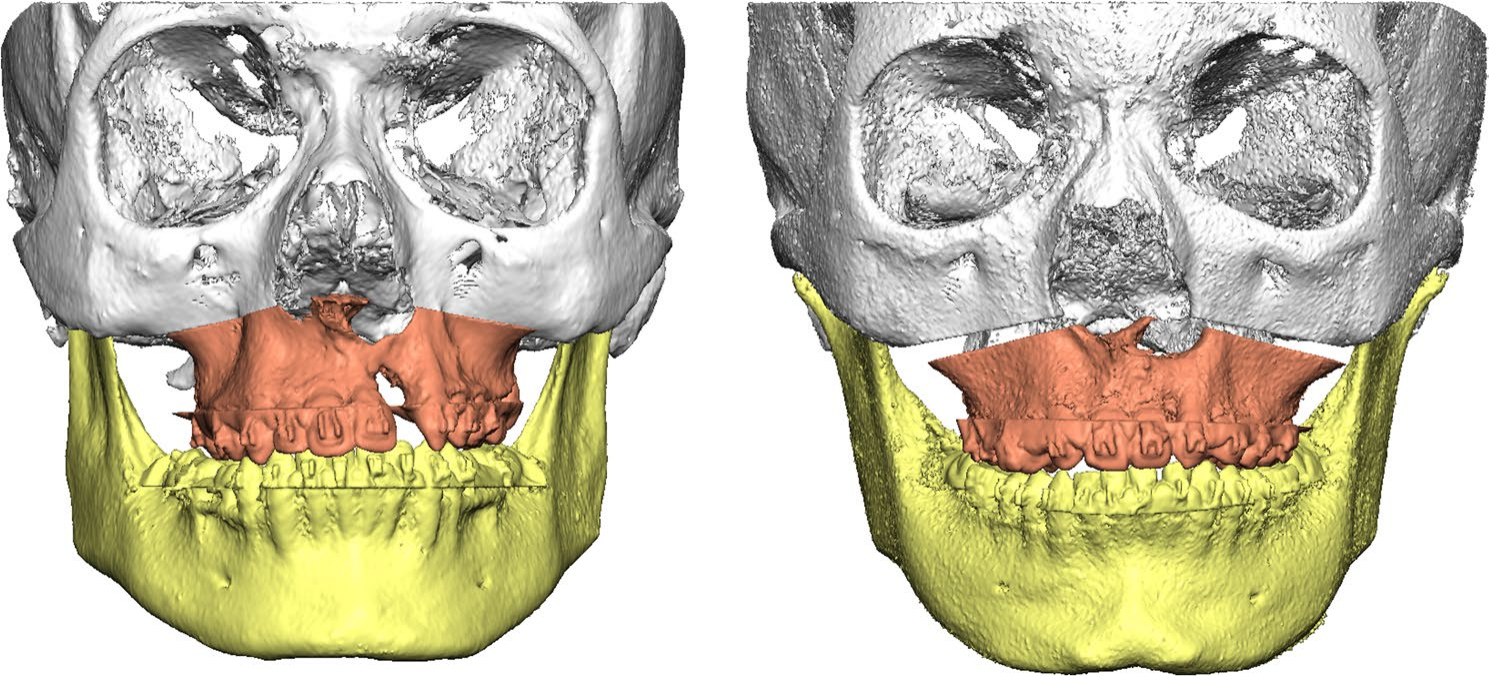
Postoperative situation, frontal view. Left: CBCT image acquired using the standard-dose FACE protocol. Right: CBCT image acquired using the ULD JAW protocol
